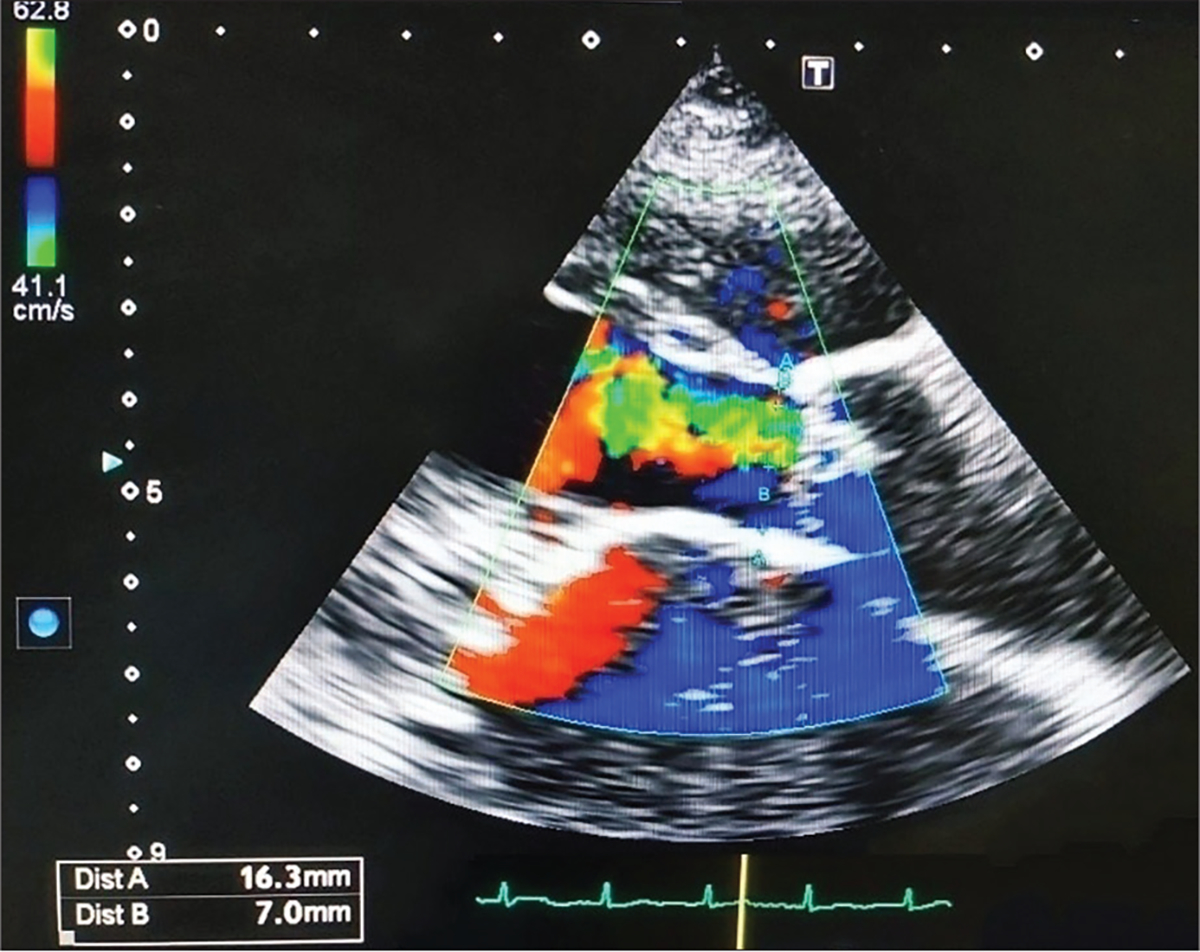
Parasternal long axis view using color Doppler flow imaging of aortic valve showing severe aortic regurgitation with vena contract width of 7 mm
